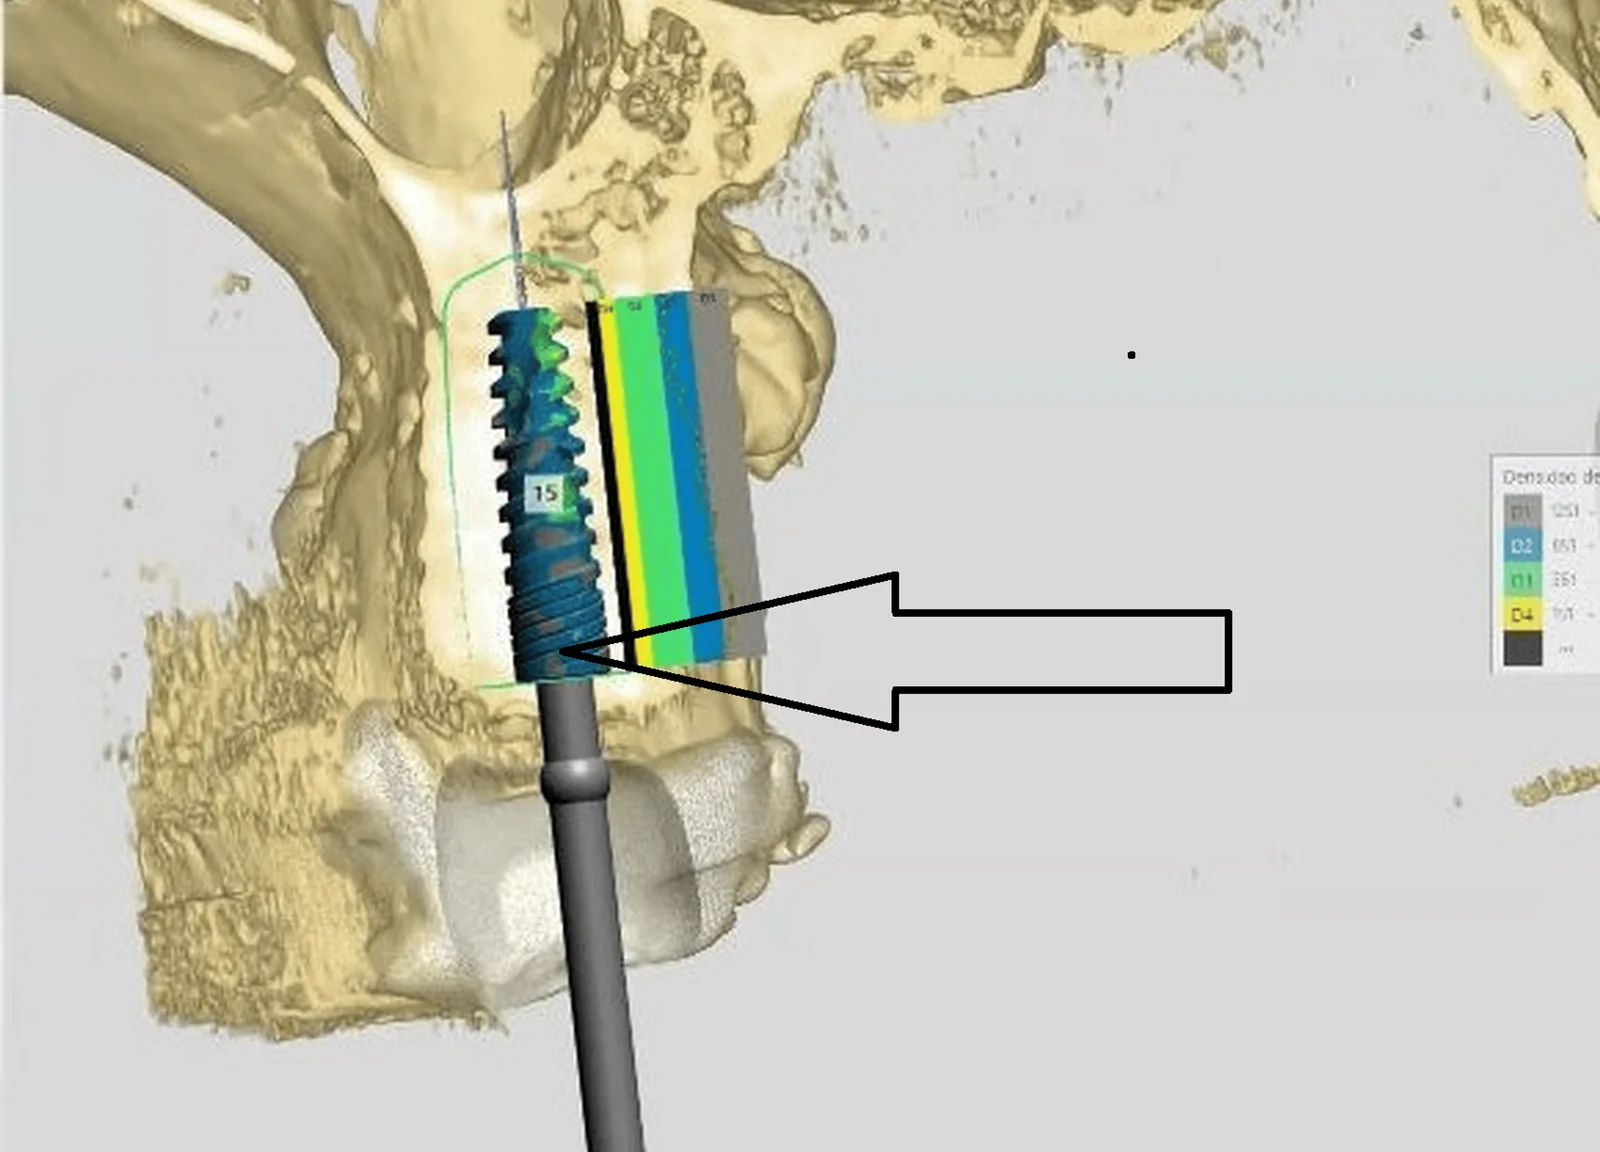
Three-dimensional CBCT-guided digital implant planning for rehabilitation of the maxillary right second premolar (tooth #4). CBCT planning was performed after alveolar ridge preservation to evaluate the regenerated bone and facilitate prosthetically driven implant placement in the maxillary right second premolar (tooth #4) site. The black arrow indicates the virtual implant positioned within the regenerated alveolar ridge, allowing assessment of implant angulation, depth, and surrounding anatomical structures before surgery. CBCT: cone-beam computed tomography
